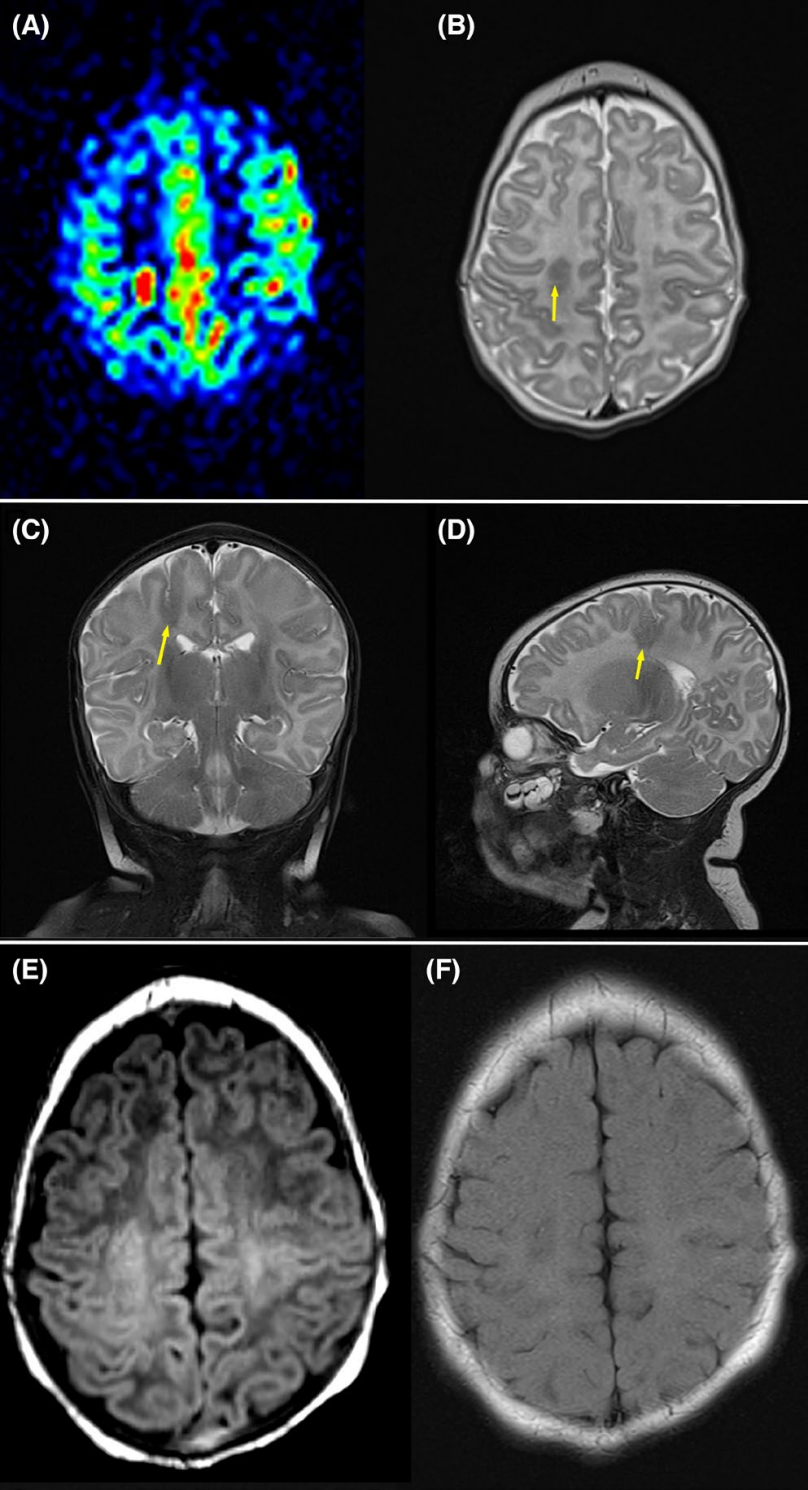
Case 1: Two‐month‐old with right posterior superior frontal bottom‐of‐sulcus FCD IIa. Note that all images in all figures are in radiological orientation. (A) Axial perfusion‐weighted pseudo‐continuous ASL MRI demonstrating hyperperfusion in the region of FCD. No ASL scale available. Slice thickness 4 mm, delay time 1030 ms, pixel size 2.9 mm^2^. (B) Axial T2 with thickening and blurring of gray‐white junction. (C) Coronal T2 with thickening and blurring of gray‐white junction. (D) Sagittal T2 with thickening and blurring of gray‐white junction. (E, F) Note that myelinated subcortical white matter on the left has a similar signal to the right FCD on axial T1 (E), making it less obvious. The FCD is not apparent on axial T2 FLAIR (F), and is most distinct on axial T2 due to better signal contrast (B).
